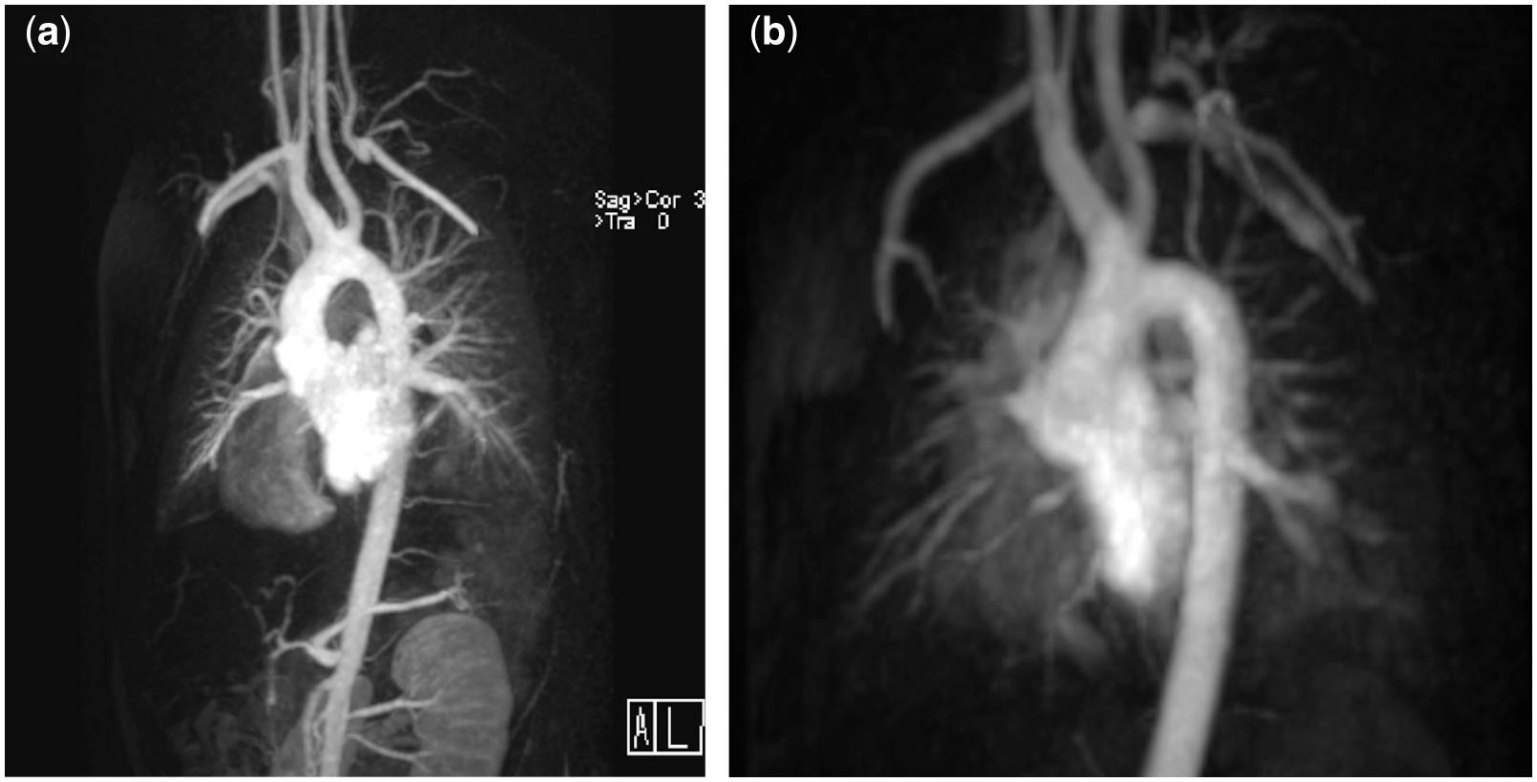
(a) Magnetic resonance imaging 15 years after direct AA anastomosis, ligation and division of the left subclavian artery and VSD closure in a patient with IAA type B, VSD and bicuspid aortic valve. (b) 11 years after Rastelli procedure, AA patch plasty (curved polytetrafluorethylene patch), ligation and division of the left subclavian artery in a patient with truncus arteriosus communis type A1 (Van Praagh) and IAA type B
